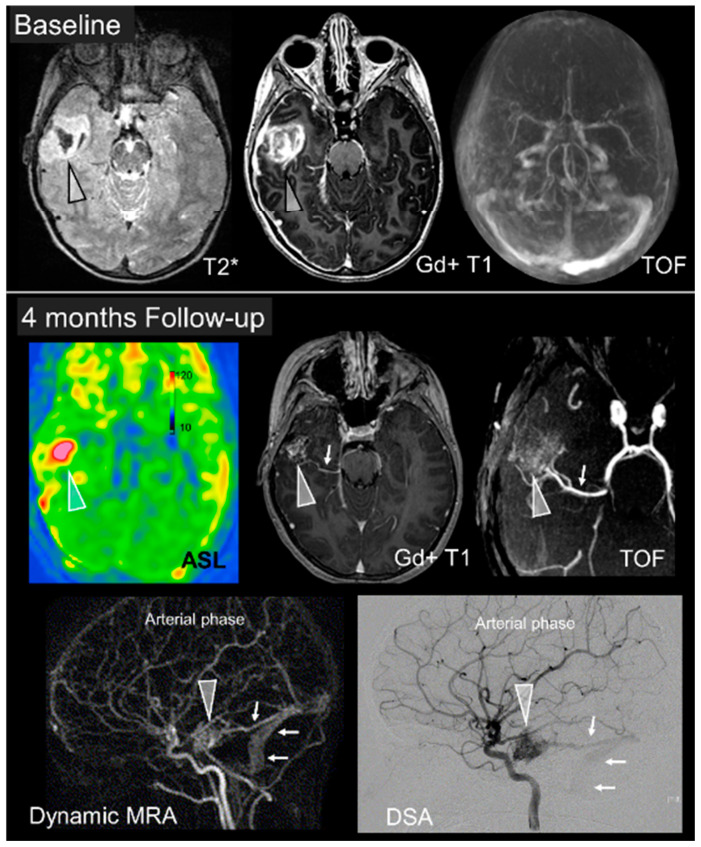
Advanced imaging. MRI images of a 5-year-old girl presenting with acute headaches and confusion. The top row shows an early subacute intracerebral hemorrhage (Arrowhead, HypotT2* and HyperT1) with no detectable vascular anomaly on the TOF sequence. DSA was also found to be normal at that time. The bottom panels show the imaging appearance 4 months thereafter; with increased cerebral blood flow (CBF) within a vascular nidus (Arrowhead) seen on the ASL sequence, post contrast T1, and TOF. Dynamic MRA shows early enhancement of cerebral veins and a nidus within the parenchyma characterizing a brain arteriovenous malformation (AVM), confirmed on the DSA acquired the next day.
